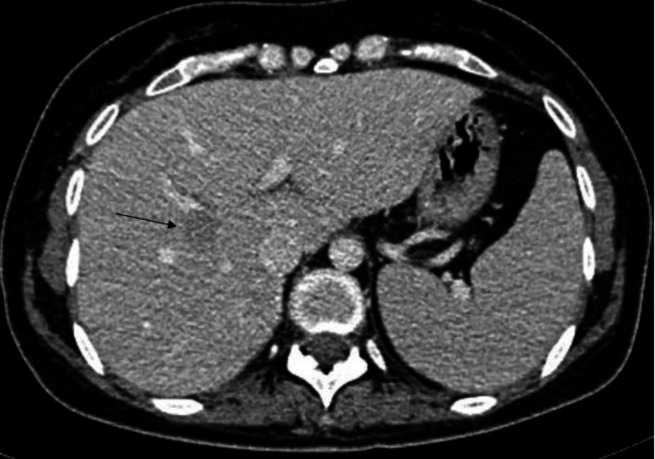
Axial CT image of the abdomen in late arterial phase for a CT-guided core biopsy denoting the hypodense lesion of segment VIII to be biopsied (arrow). To note intravenous contrast was administered prior to the CT-guided biopsy for better visualization of the previously described hepatic lesions under investigation
